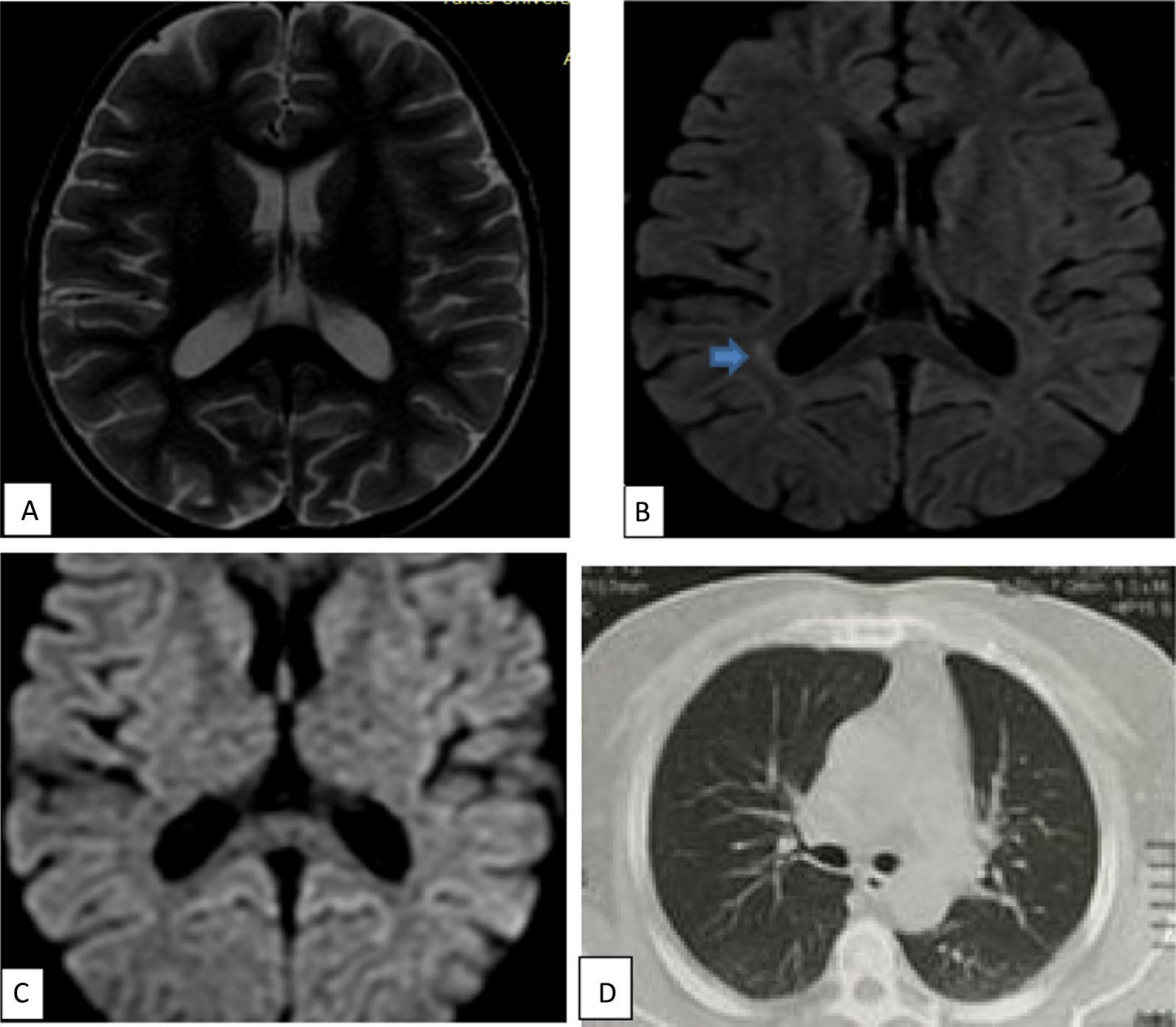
A 17-year-old female patient with chronic kidney disease presented with post COVID puerperal skin rash, headache and decreased conscious level. MRI axial T2WI (a) hardly detect abnormal signal and axial FLAIR (b) revealed abnormal foci of increased signal intensity in right periventricular deep white matter (blue arrows). Diffusion images (c) show no restricted diffusion. Post COVID vasculitis was considered. CT chest during acute phase not shown. CT chest at time of admission show no abnormalities (CORAD-I)
